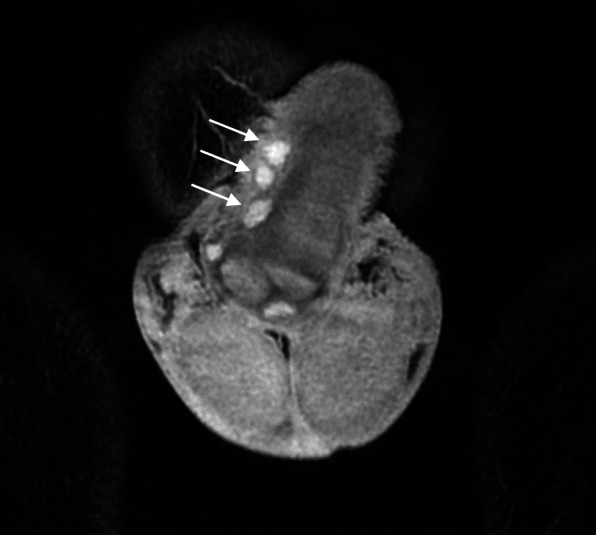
Coronal T1-weighted post contrast MRI of penile nodules. Coronal T1-weighted post contrast MRI showing penile nodules of low signal intensity with homogenous and avid post contrast enhancement (arrows)
